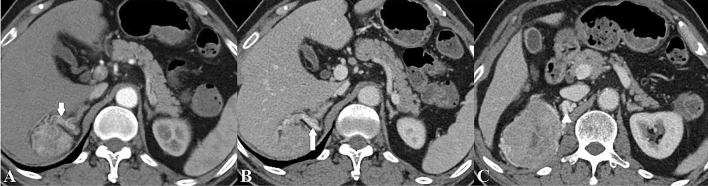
A 70-year-old man with a 7.3 cm clear cell carcinoma in the right kidney. Contrast-enhanced computed tomographic images demonstrate a prominent peritumoral collateral artery (short arrow) during the corticomedullary phase (A) and collateral vein (long arrow) during the nephrographic phase (B) in the right perinephric space. The collateral vein during the nephrographic phase (C) measures 0.5 cm in diameter (arrowhead). The collateral artery originates from the right adrenal artery, and the collateral vein is present in the form of inferior vena cava.
